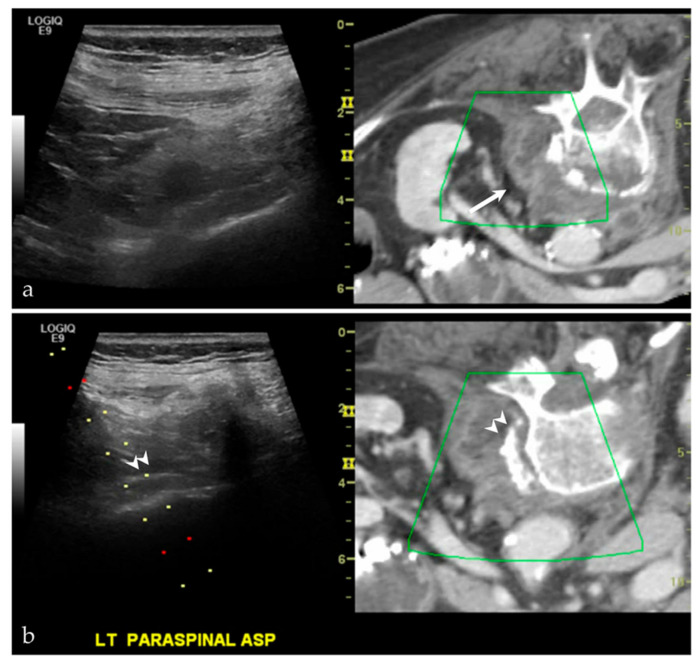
A middle-aged female with back pain, known psoas abscess. The initial images (a) show that the patient’s known abscess, seen on the fused CT images (white arrow), is hard to see on ultrasound alone. Using VNav, and using the disc-osteophyte complex as a guide (white arrowhead), it was easy to target the area of abscess/phlegmonous change (b). Aspiration was successful, yielding purulent material. Please note, the green boxes in both (a,b) are guides for the fusion software. The dotted lines in (b) are part of a targeted needle biopsy tract/approach.
